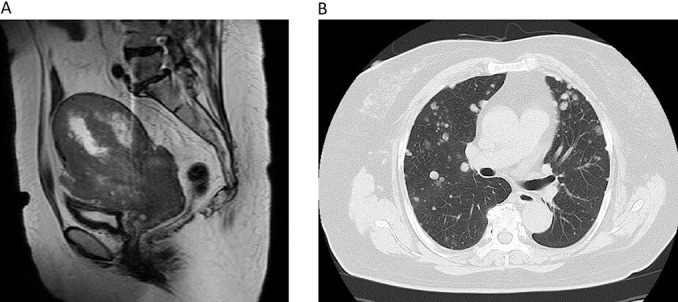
A: Plain MR image of the pelvis before treatment (T2-weighted image): A low-signal-intensity tumor occupying the uterine cervix to corpus was observed. In the inner area of the tumor, hemorrhage was noted. On the anterior wall side, infiltration beyond the serous membrane was suspected. B: Thoracic CT image: In the bilateral lung fields, masses suggestive of multiple metastases were observed.
